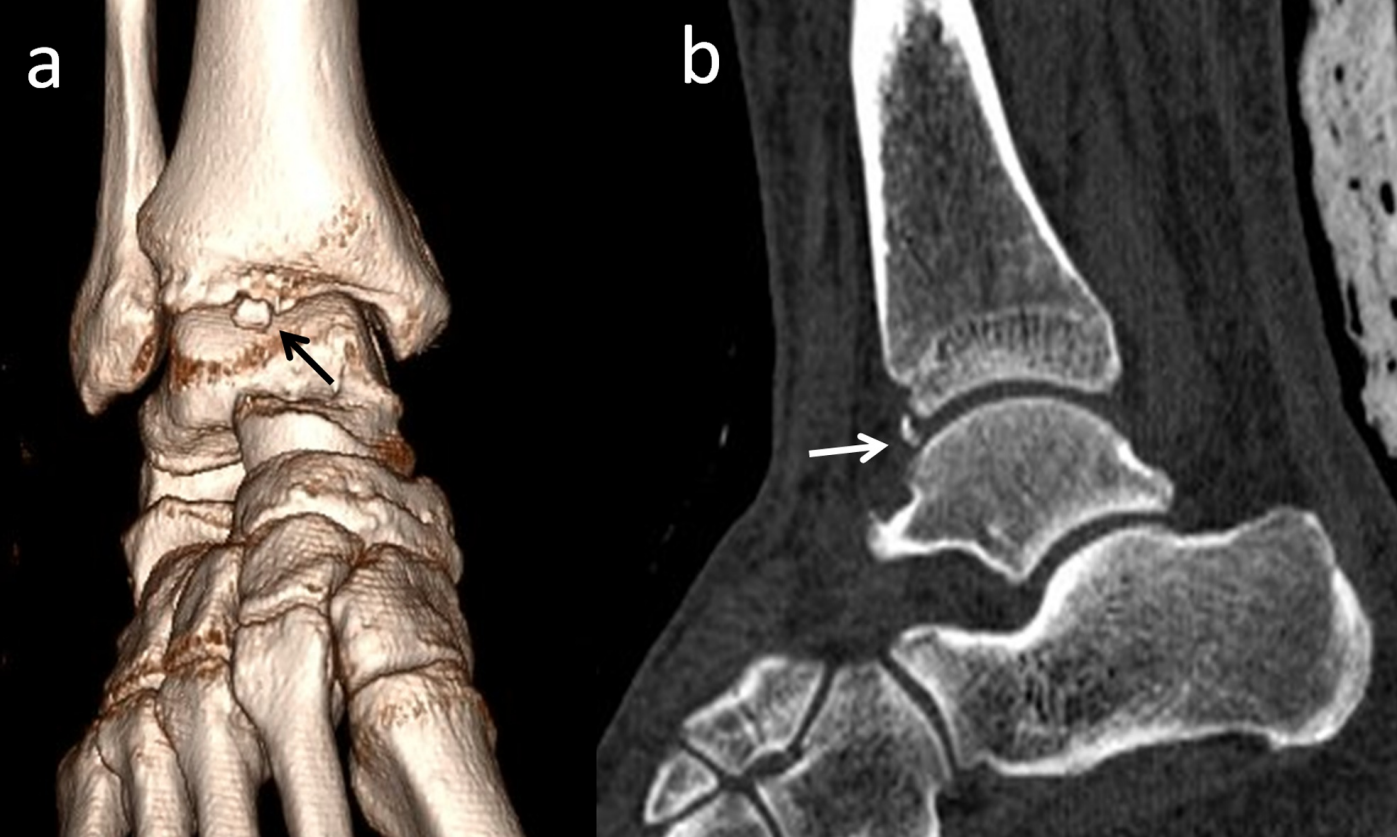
A patient with anterior ankle impingement syndrome (a) 3D computed tomography (CT) appearance, the black arrow shows the os talotibiale; (b) 2D CT appearance, the white arrow shows the os talotibiale.
